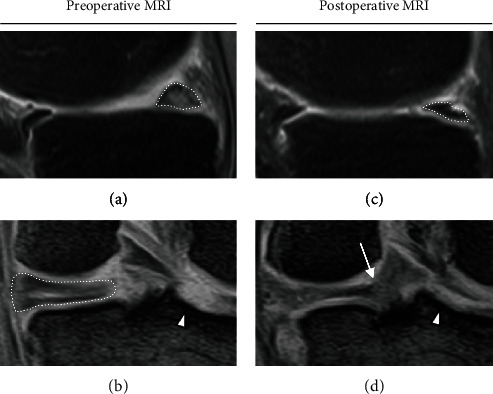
Magnetic resonance imaging. (a) Ghost sign (dotted area) on sagittal view. (b) Giraffe neck sign (dotted area) and highly damaged articular cartilage on the lateral tibial plateau beside the lateral tibial eminence (arrowhead) on coronal view. (c) Repaired medial meniscus (MM) root (dotted area). (d) Continuity of the repaired MM root (arrow) and improved articular cartilage (arrowhead).
